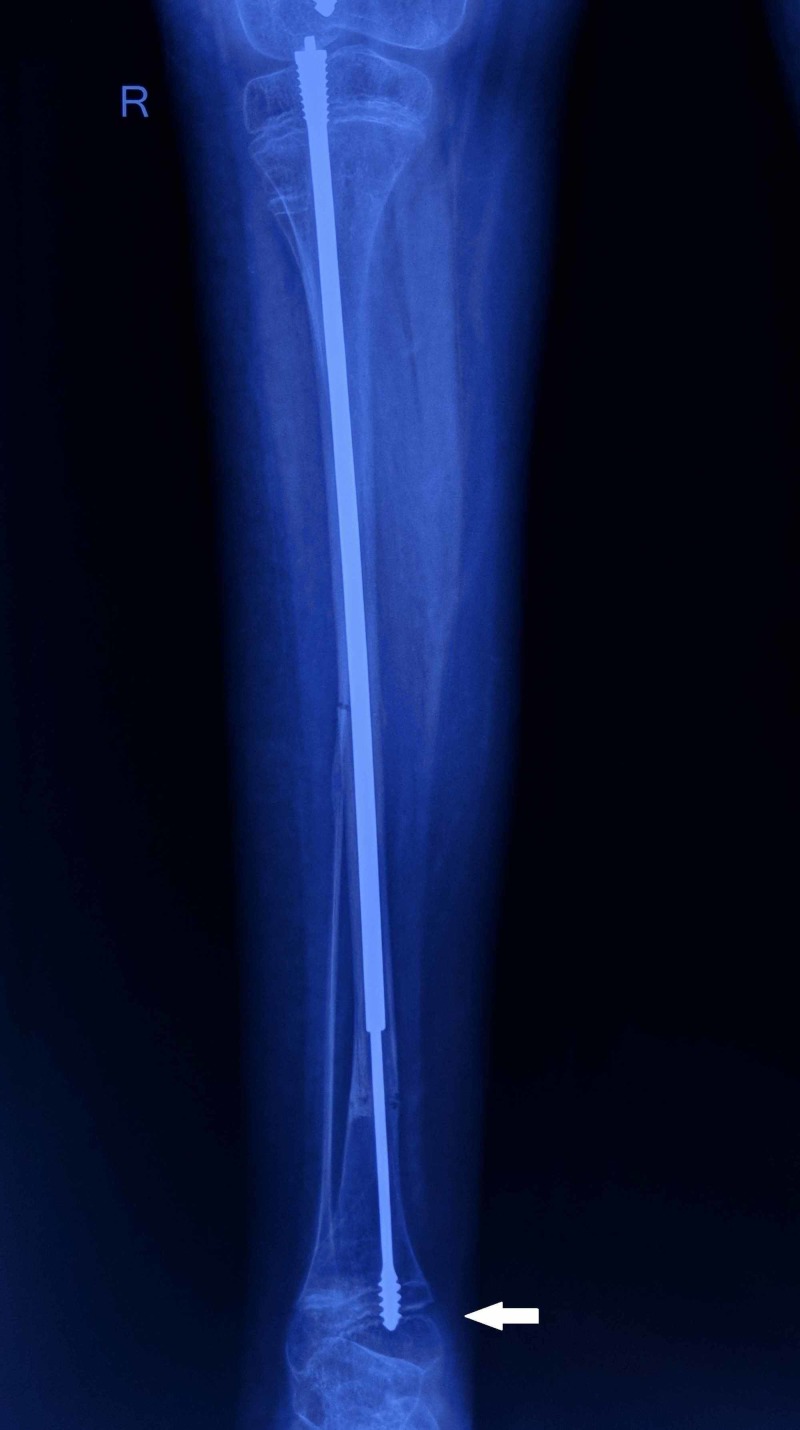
Follow-up radiograph of the right tibia The image shows the male component of the nail getting dislodged (white arrow) from the epiphysis
